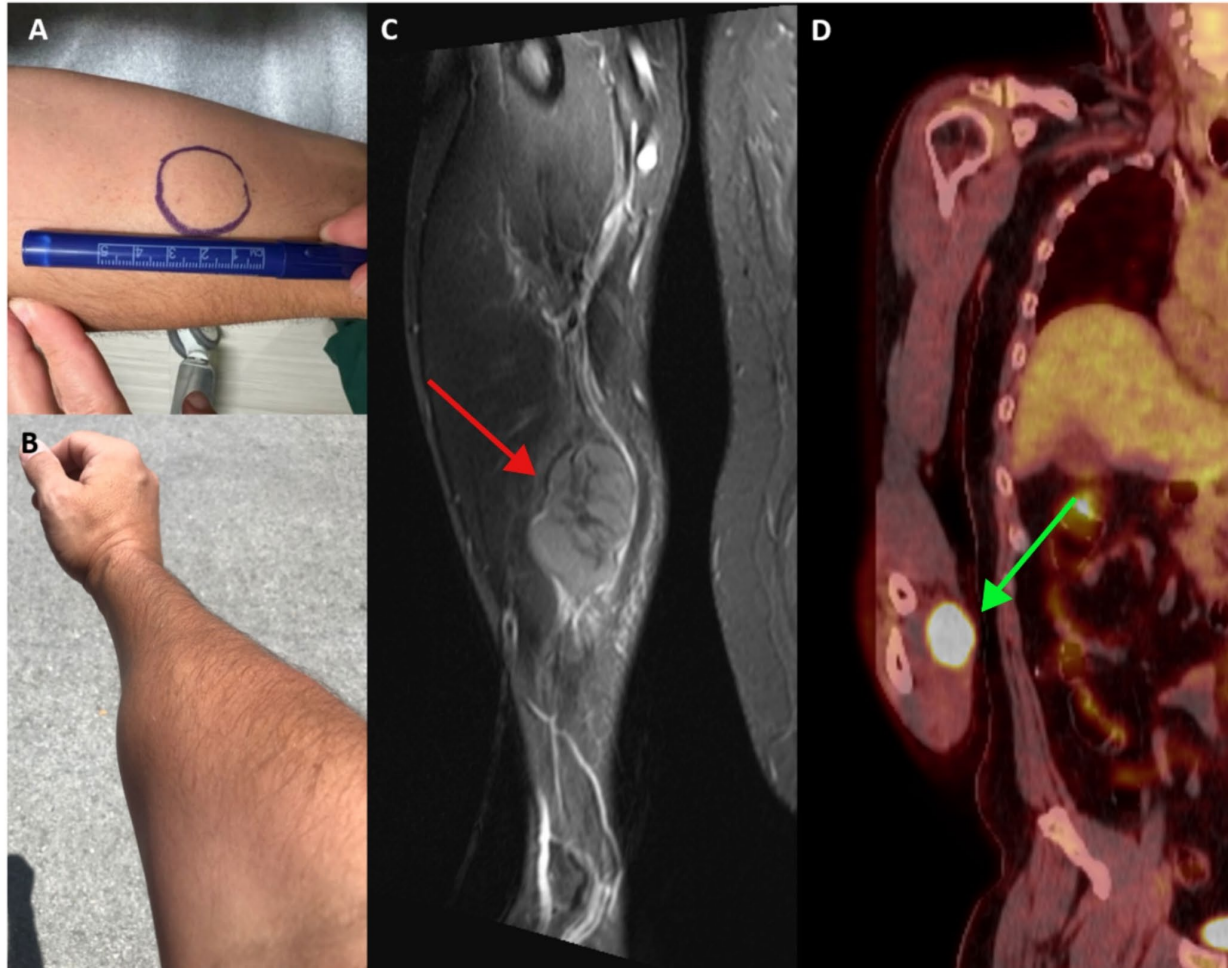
Case 2: Appearance of the soft tissue forearm lesion on the patient’s arm when it initially came to attention (A) and after lesion growth (B); C) MRI with contrast of right ulna and radius demonstrates intermuscular soft tissue mass within the volar and radial aspect of the mid third of the forearm, measuring 6.0 × 3.2 × 3.9 cm (CC X AP X mediolateral), with a hypo-T1/hypo-T2 non-enhancing central area (red arrow). The soft tissue mass is Fludeoxyglucose F18 (FDG)-avid on PET-CT (green arrow, D)
